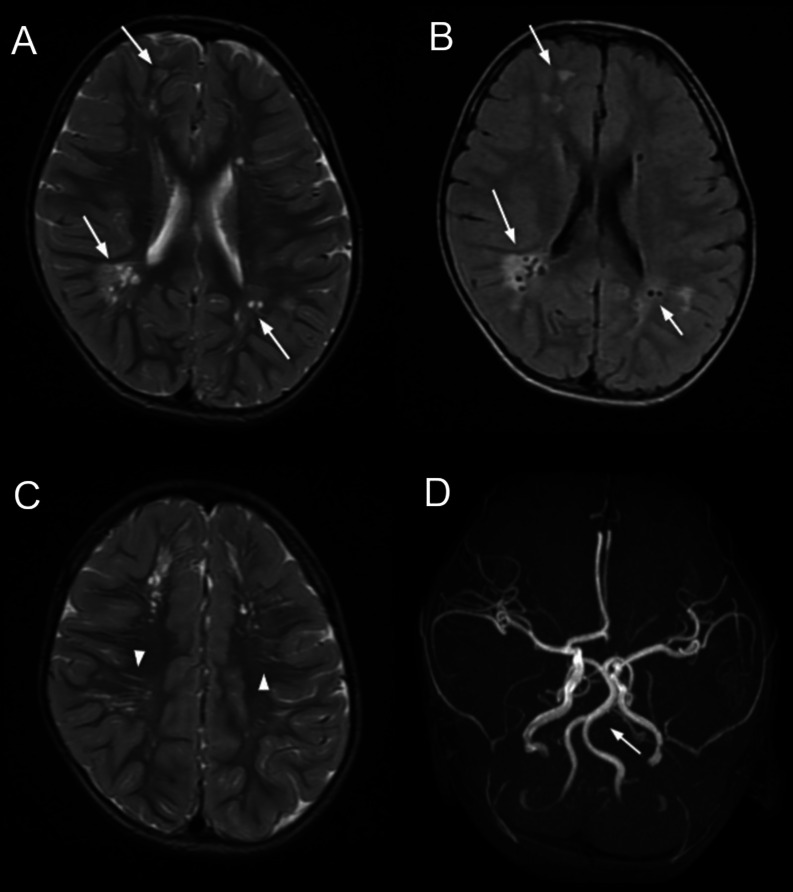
Magnetic resonance imaging of the patient at 2 years of age. On the axial T2-weighted (A) and fluid-attenuated inversion recovery (B) images, multifocal cerebral white matter lesions are visible (arrows). The T2-weighted image shows hyperintense thin stripes suggesting dilated perivascular spaces (C, arrowheads). Magnetic resonance angiography revealed vertebrobasilar artery dolichoectasia (D, arrow).
